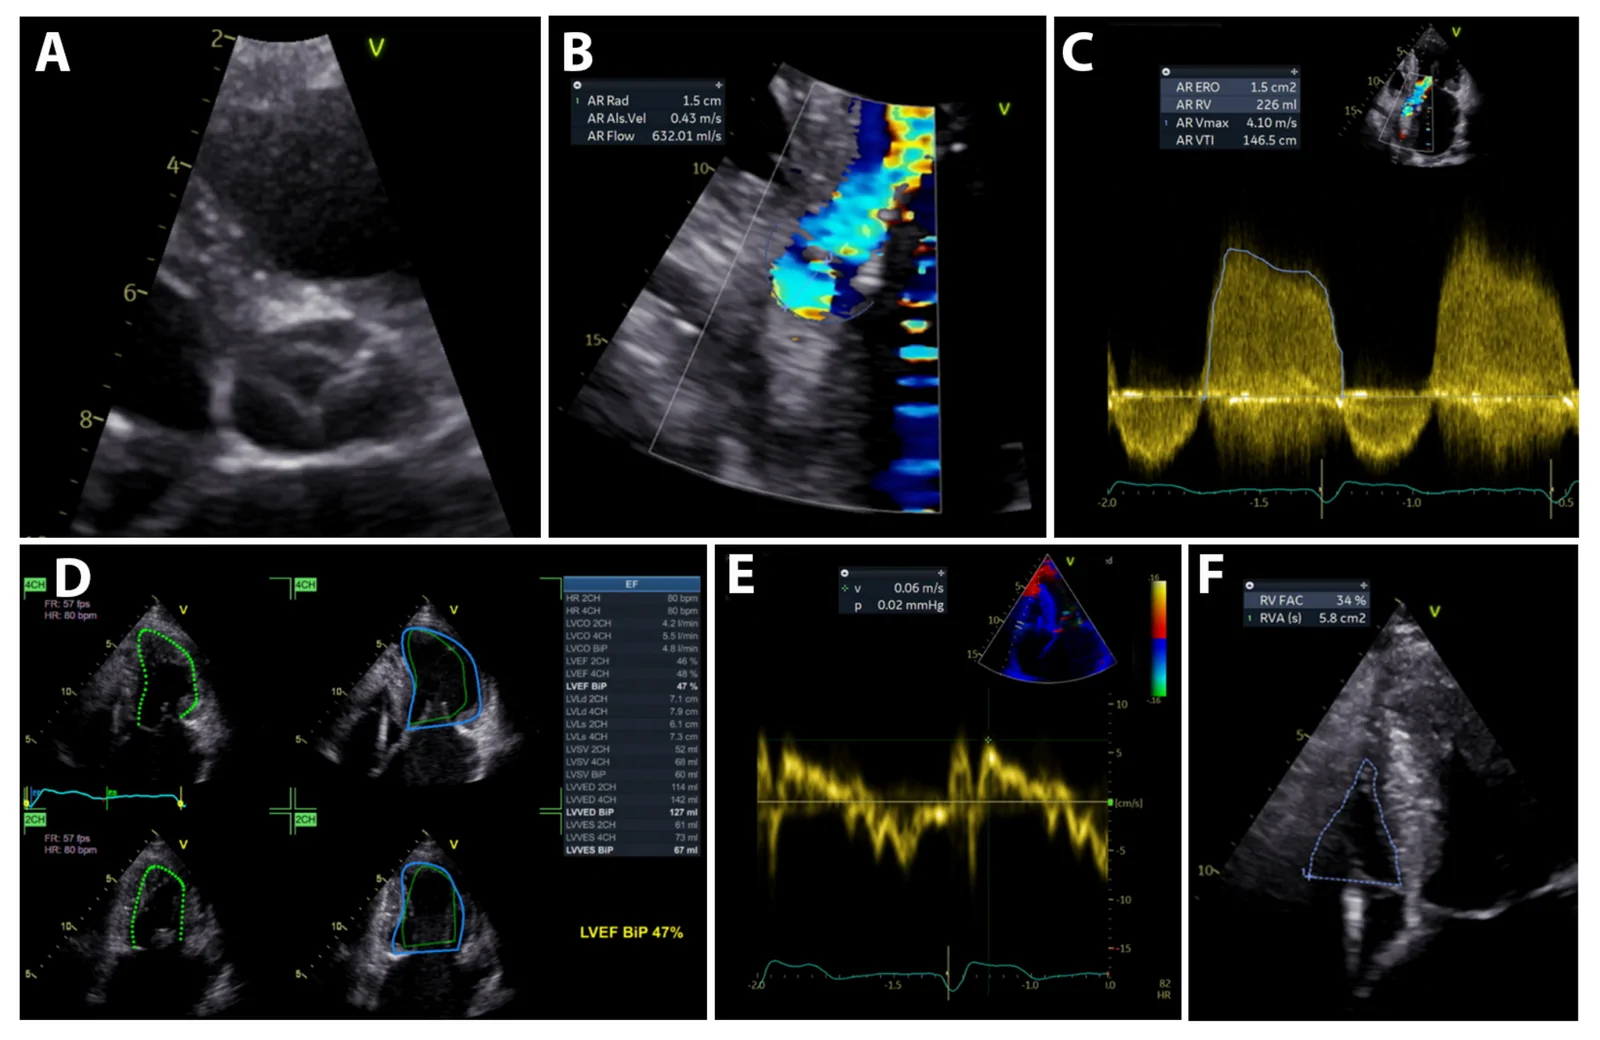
Transthoracic echocardiography showing modified tricuspid aortic valve with right cusp restriction (A) and severe regurgitation (B,C); Significant left ventricle dilatation with reduced ejection fraction (D) and severe right ventricular dysfunction (E,F).
